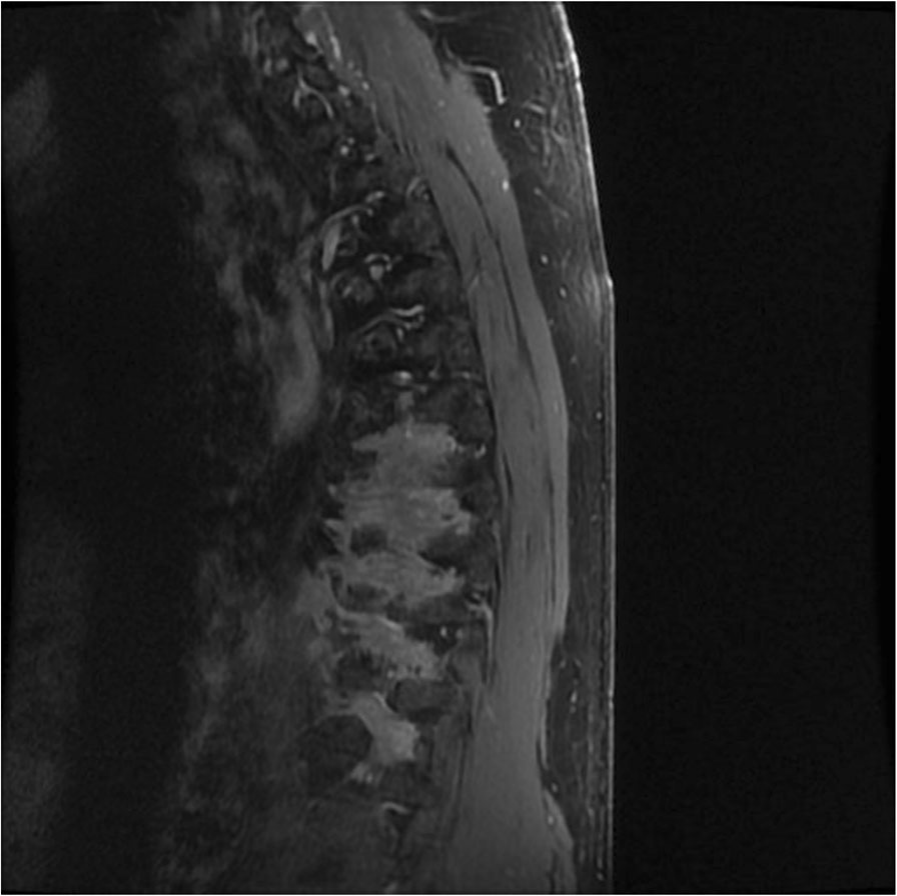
Sagittal magnetic resonance imaging of the thoracic spine. T1-weighted image with gadolinium enhancement displaying abnormal right paravertebral soft tissue thickening and enhancement along multiple thoracic levels
